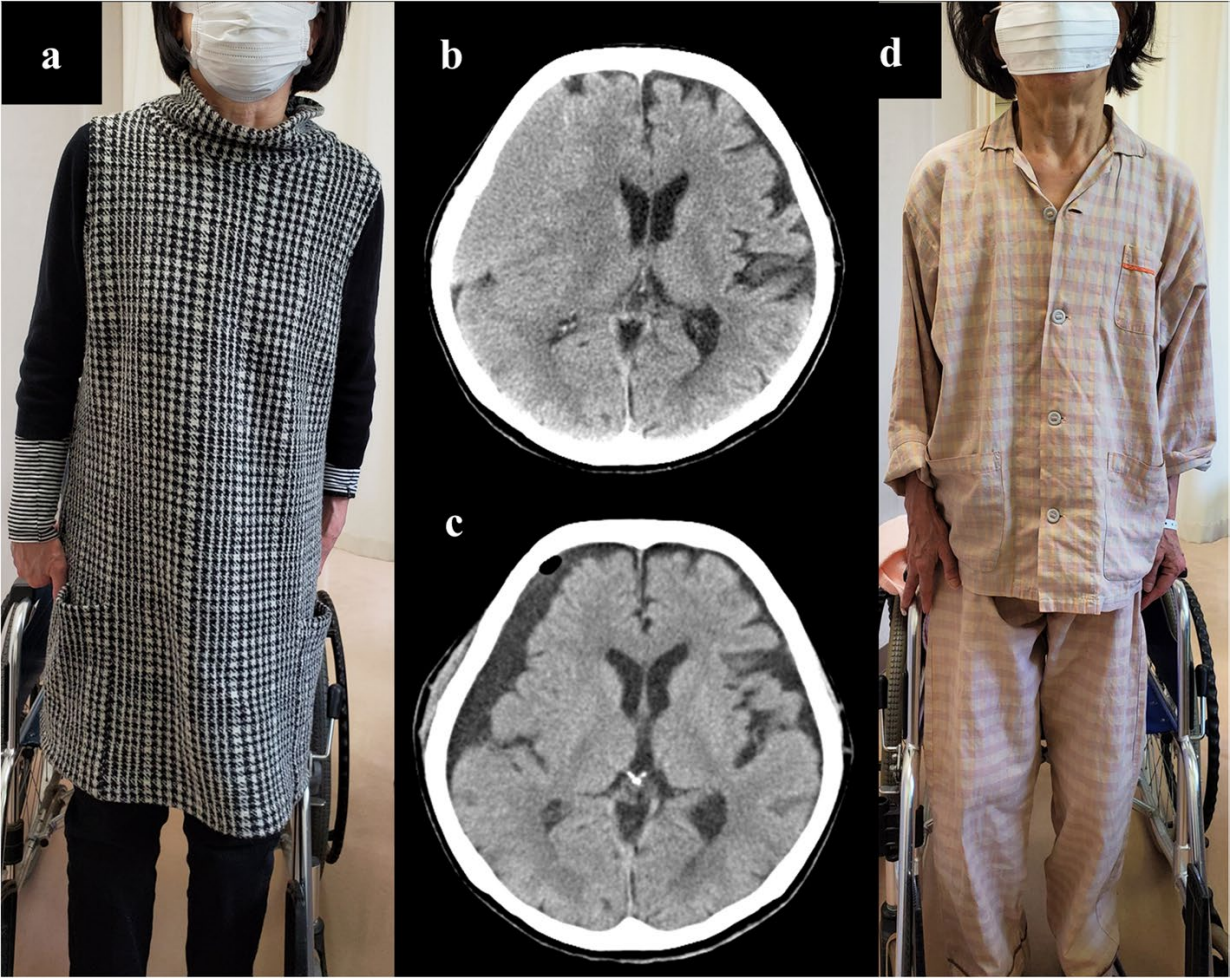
Tonic flexion of the trunk to the left was present in the standing position before surgical intervention (a). Presurgical brain CT showing voluminous subdural hematoma in the right convexity with marked compression of the right hemisphere (b). Postsurgical brain CT carried out four days after the operation showing improvement of the right subdural hematoma (c). Seven days after hematoma evacuation, tonic flexion of the trunk disappeared (d)
